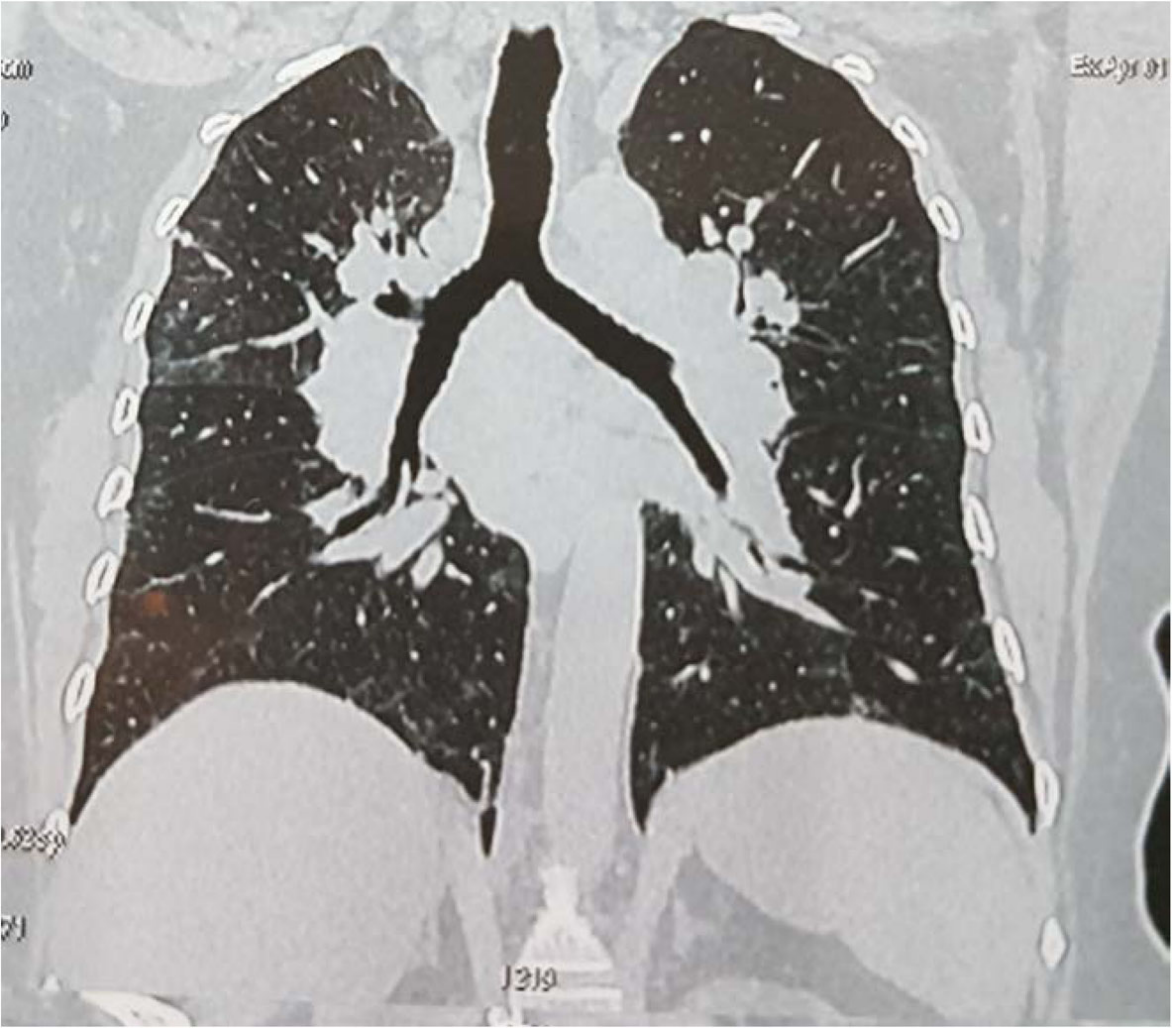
Coronal section of HRCT chest of Eales' showing tree bud appearance of the enlarged hilar lymph nodes
